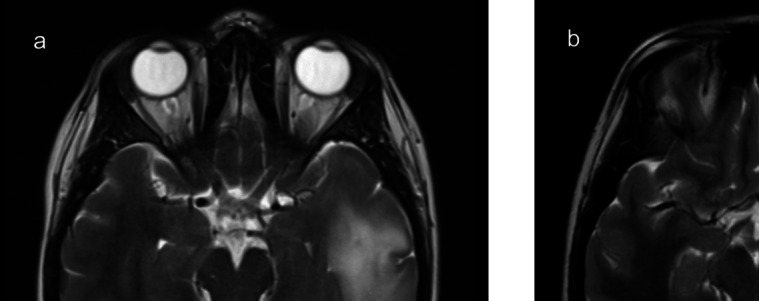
Case 1 – Preoperative MRI (axial view) demonstrating a left-temporal tumour with anterior structures highlighted (a). Post-operative MRI confirming complete resection (b)
